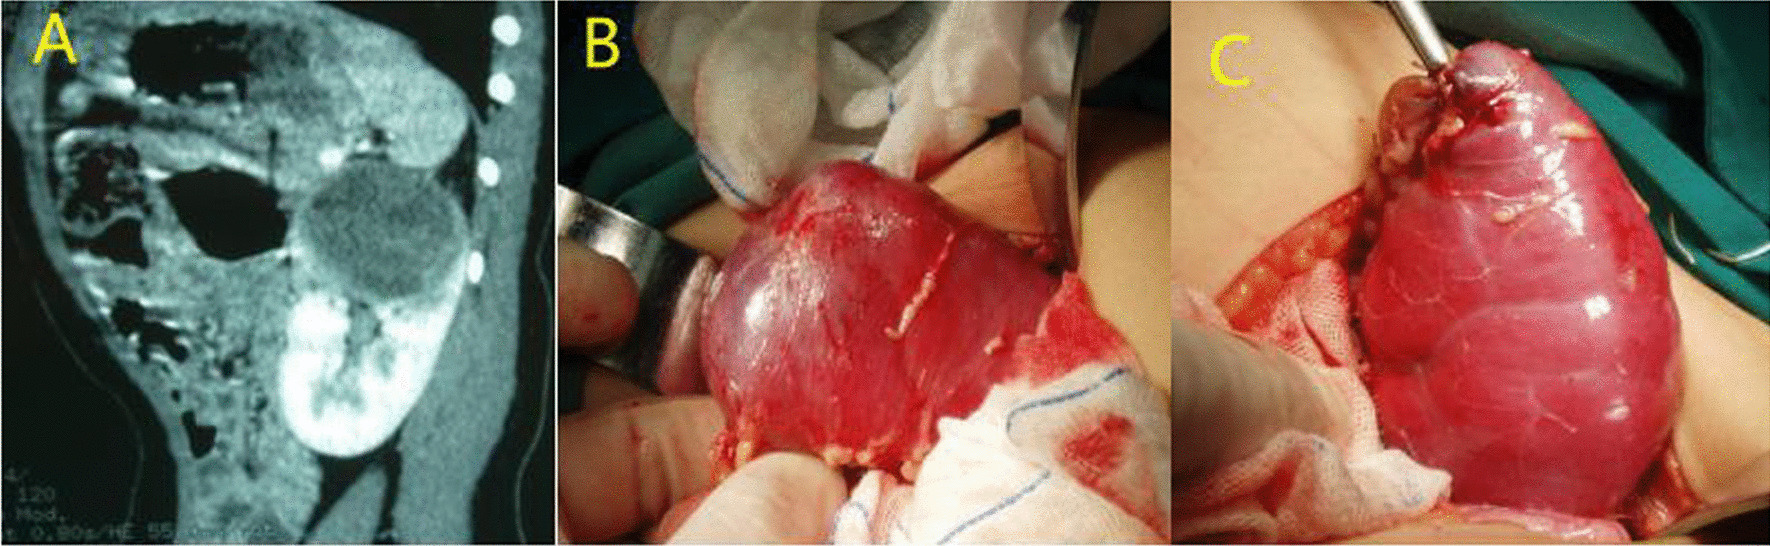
NSS performed in MRTK which the tumor located in the upper pole of left kidney. A The upper pole mass of the left kidney was seen in CT. B The tumor in the upper pole of the left kidney. C The left kidney after NSS
